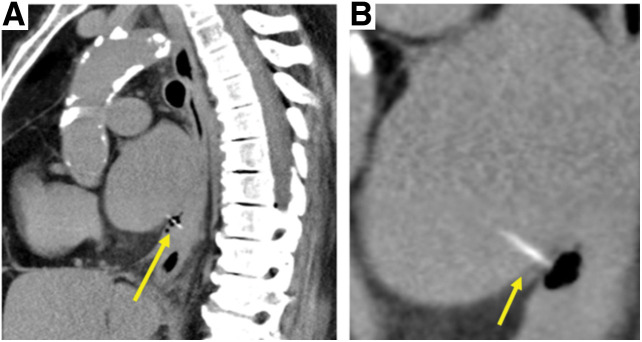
The first CT scan at our hospital. (B) is a magnified view of (A). A 2.5-cm-long, linear, hyperabsorptive structure penetrating the left atrium from the ventral side of the lower thoracic esophagus is observed (yellow arrows). Free air and fluid collection are also observed in the lower mediastinum.
